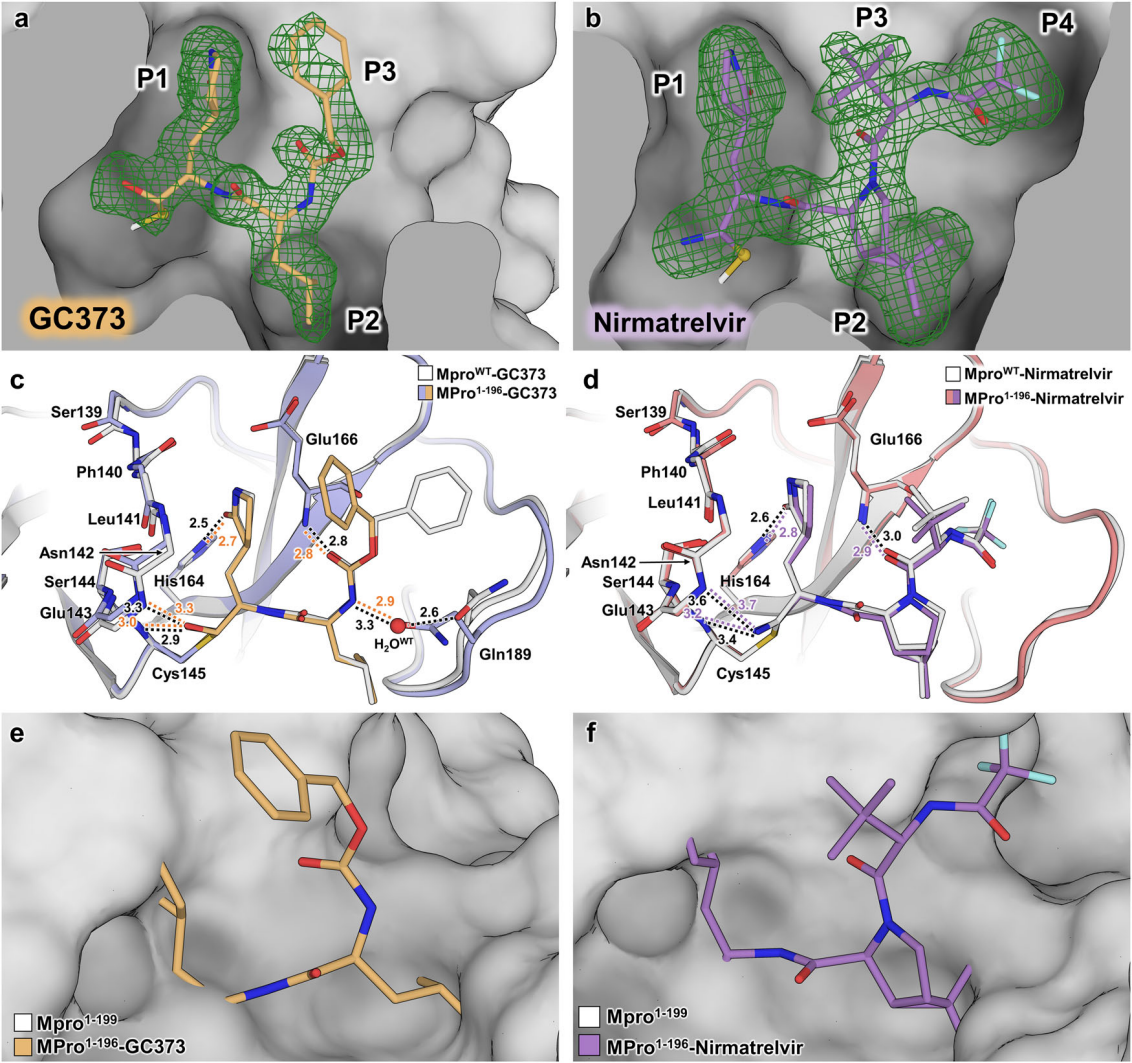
Room-temperature X-ray structures of MPro^1–196^ in complex with covalent inhibitors GC373 and NMV. a, b Electron density of GC373 (orange sticks) and NMV (purple sticks) in the active site of MPro^1–196^ molecule A shown as Polder omit maps (green mesh contoured at 3σ). c, d Superposition of MPro^WT^-inhibitor complex monomer with the equivalent MPro^1–196^-inhibitor complex (molecule B). The main chain of oxyanion loop residues 139–145 are shown creating the oxyanion hole and interacting with ligand. Hydrogen bonds represented as color-coded dashes with distances in Å. All distances are shown in Å. e, f Superposition of inhibitor-free MPro^1–199^ with the GC373 and NMV MPro^1–196^ complex, respectively. Inhibitor-free MPro^1–199^ represented as white surface where the conformational shifts described result in an occluded ligand-binding site. All superpositions performed as least-squares fit of Cα residues modeled in MPro^1–199^.
